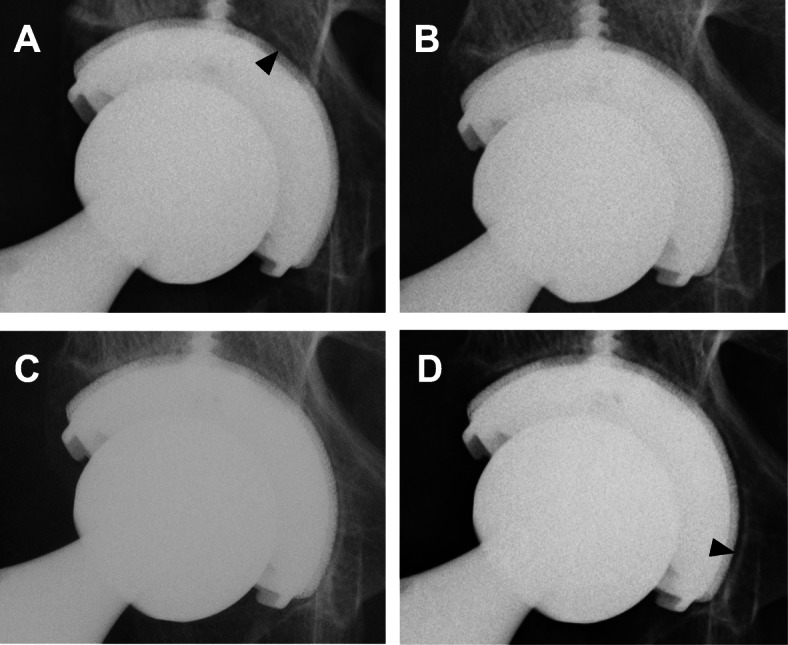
Plain radiographs of the same patient as in Fig. 2. a At 1 week after surgery, there was an RL (black arrowhead) in zone 2. b At 6 months after surgery, there was no RL. c At 12 months after surgery, there were no RLs ≥ 1 mm as considered significant. d At 2 years after surgery, there was an RL (black arrowhead) in zone 3
